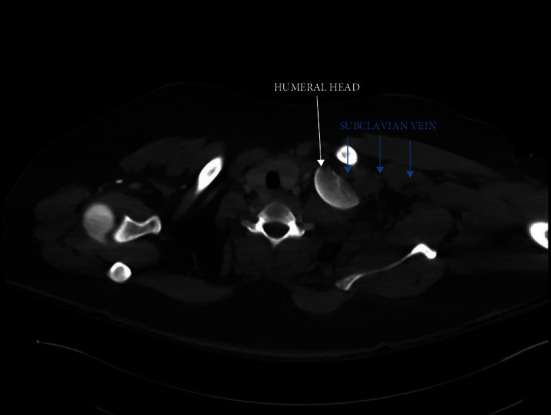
Preoperative CT scan of the chest with contrast confirms 4-part fracture of the proximal humerus with sheared off humeral head displaced posterior to the medial left clavicle above the first rib. The left subclavian vein is attenuated.
